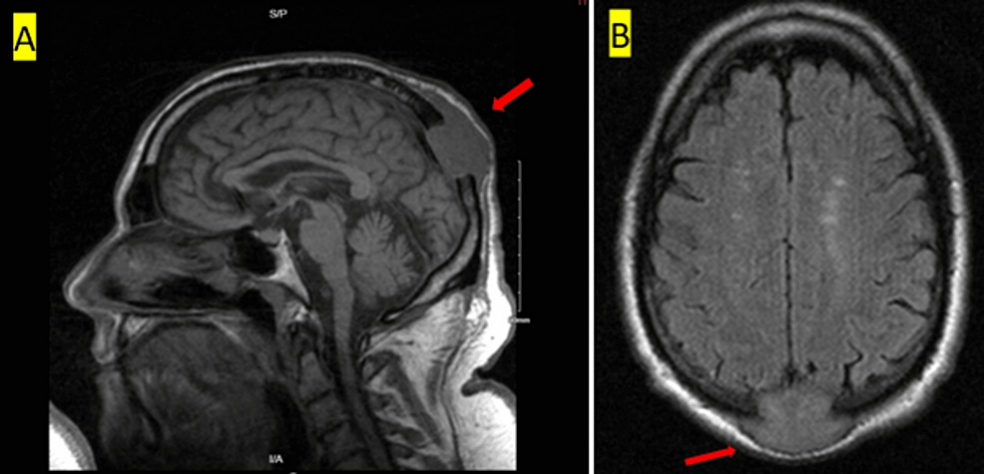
MRI brain without contrast (A) Anterior displacement of the superior sagittal sinus, without evidence of sinus invasion. (B) 5 cm lytic mass centered in the posterior midline parietal and occipital bone eroding through the inner and outer table. Mild extension into the epidural space with mild mass effect on the parietal lobe.
